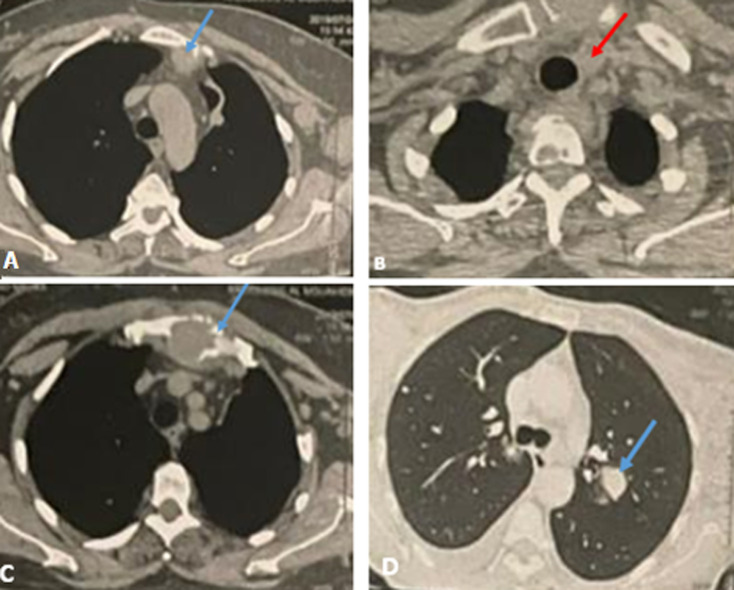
chest CT scan, mediastinal window (A, B, C), axial slices (A, B) showing adenopathies of the prevascular territory (blue arrow) and upper left laterotracheal territory (red arrow), osteolytic lesion of the sternum (C) (blue arrow), parenchymal window, axial slice showing a left apex dense nodule (blue arrow) (D)
